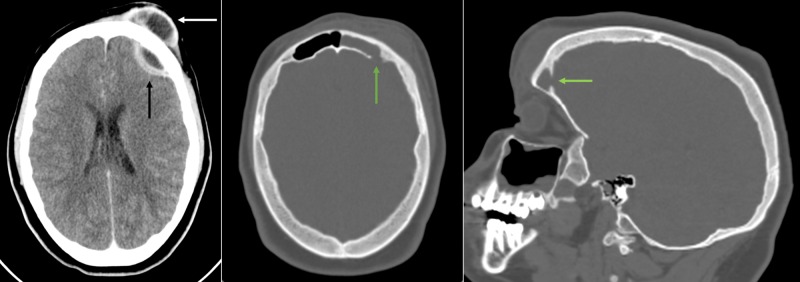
Preoperative CT scans of the head Left: Contrast-enhanced axial computed tomography (CT) scan with epidural (black arrow) and frontal periosteal (white arrow) enhancing mass compatible with an abscess. Middle: Note the bony erosion of the internal wall of the frontal sinus (green arrow) seen on the axial bone window CT scan. Right: Parasagittal imaging of the bony erosion (green arrow) seen in the middle scan.
